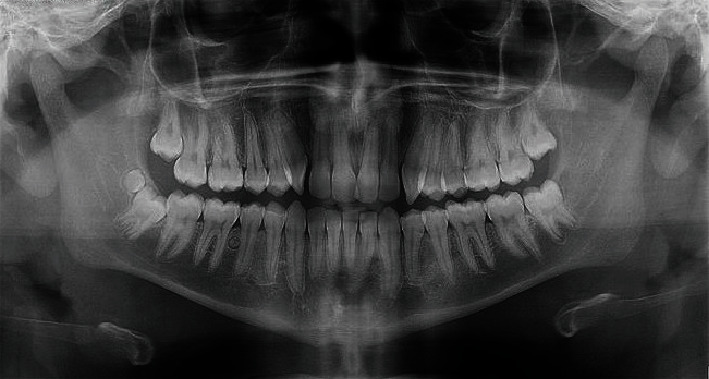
The panoramic radiograph taken after extracting 9 supernumerary teeth (all except the distomolar).
